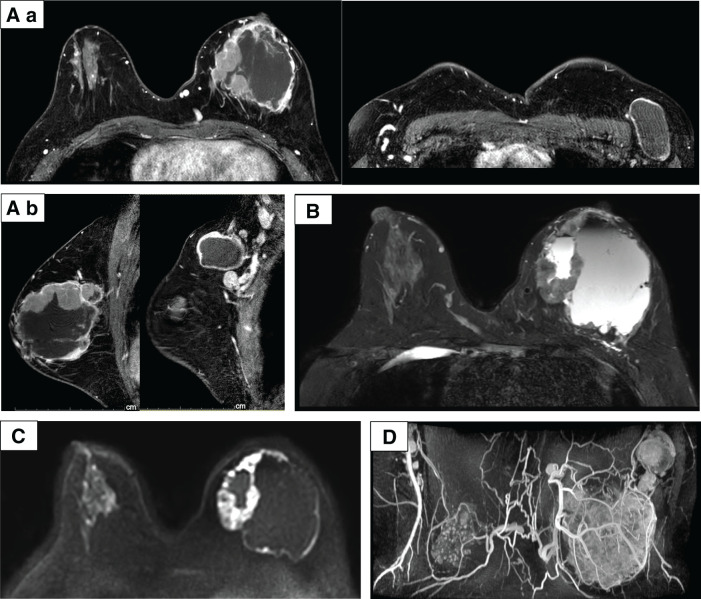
Contrast-enhanced MRI. (A) T1 (enhanced), rapid phase: (a) horizontal section and (b) sagittal section. (B) T2, (C) DWI, and (D) MIP. These findings suggest a mixed internal tumor, skin edema, and lymph node metastasis. DWI, diffusion-weighted imaging; MIP, maximum intensity projection
